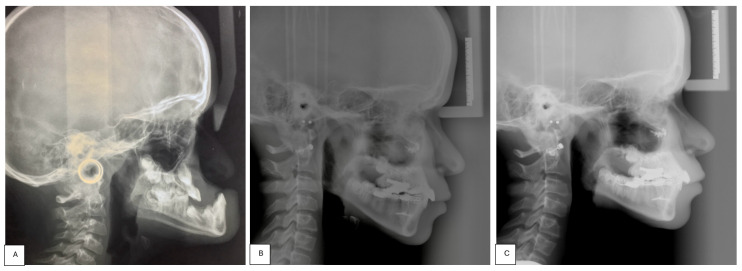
Pre-treatment (A), post-treatment (B), and 1-year follow-up (C) lateral cephalometric radiographs.
